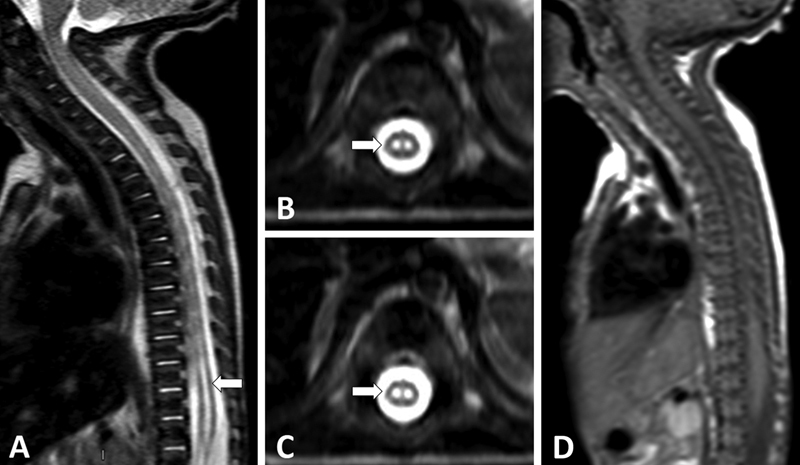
Spinal cord magnetic resonance imaging (MRI) performed at 17 days of age. Sagittal T2-weighted imaging (T2WI) shows a diffuse pencil-like hyperintense signal from D1 to the conus medullaris ((A)), and axial T2WI shows symmetric circular-ovoid foci of high signals located at the anterior horns ((B) and (C)), consistent with an “owl's eye” pattern. In its turn, sagittal T1WI ((D)) was unremarkable.
